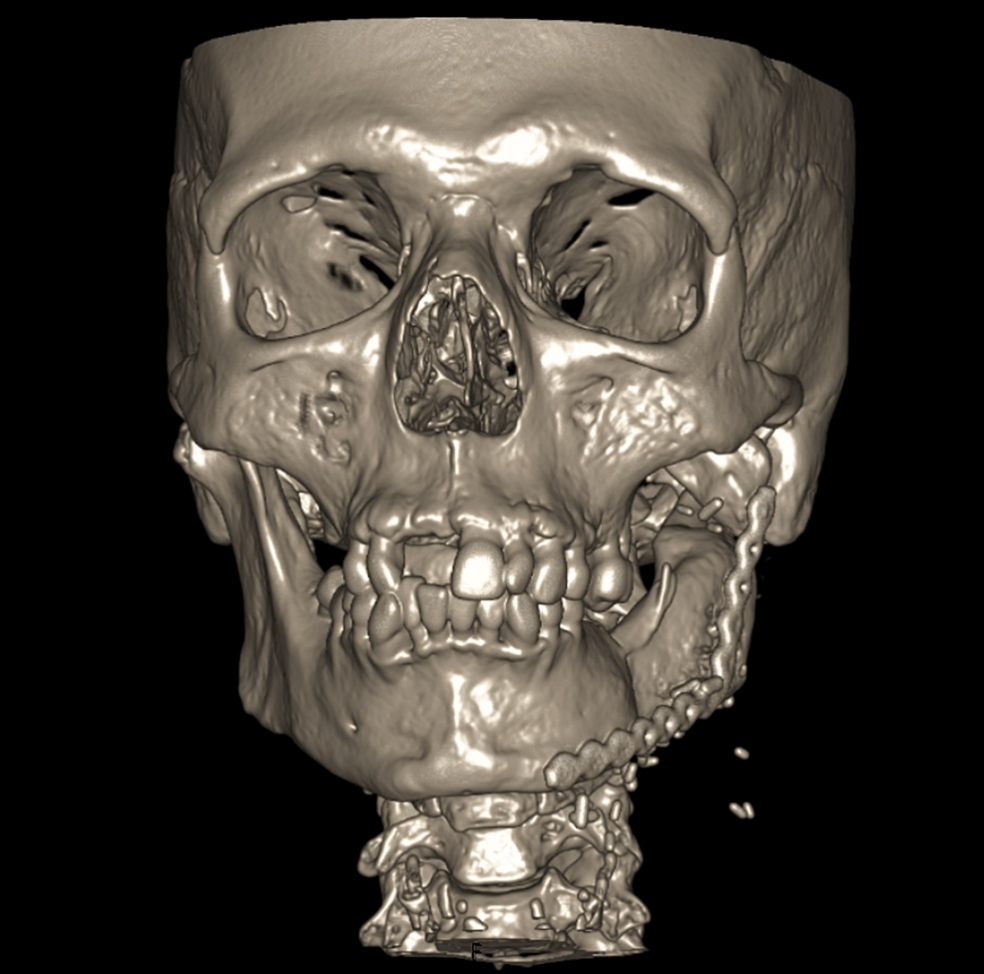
CT bone window with 3D reconstruction Displays mandibular reconstruction using a free iliac crest flap after ameloblastoma resection.
